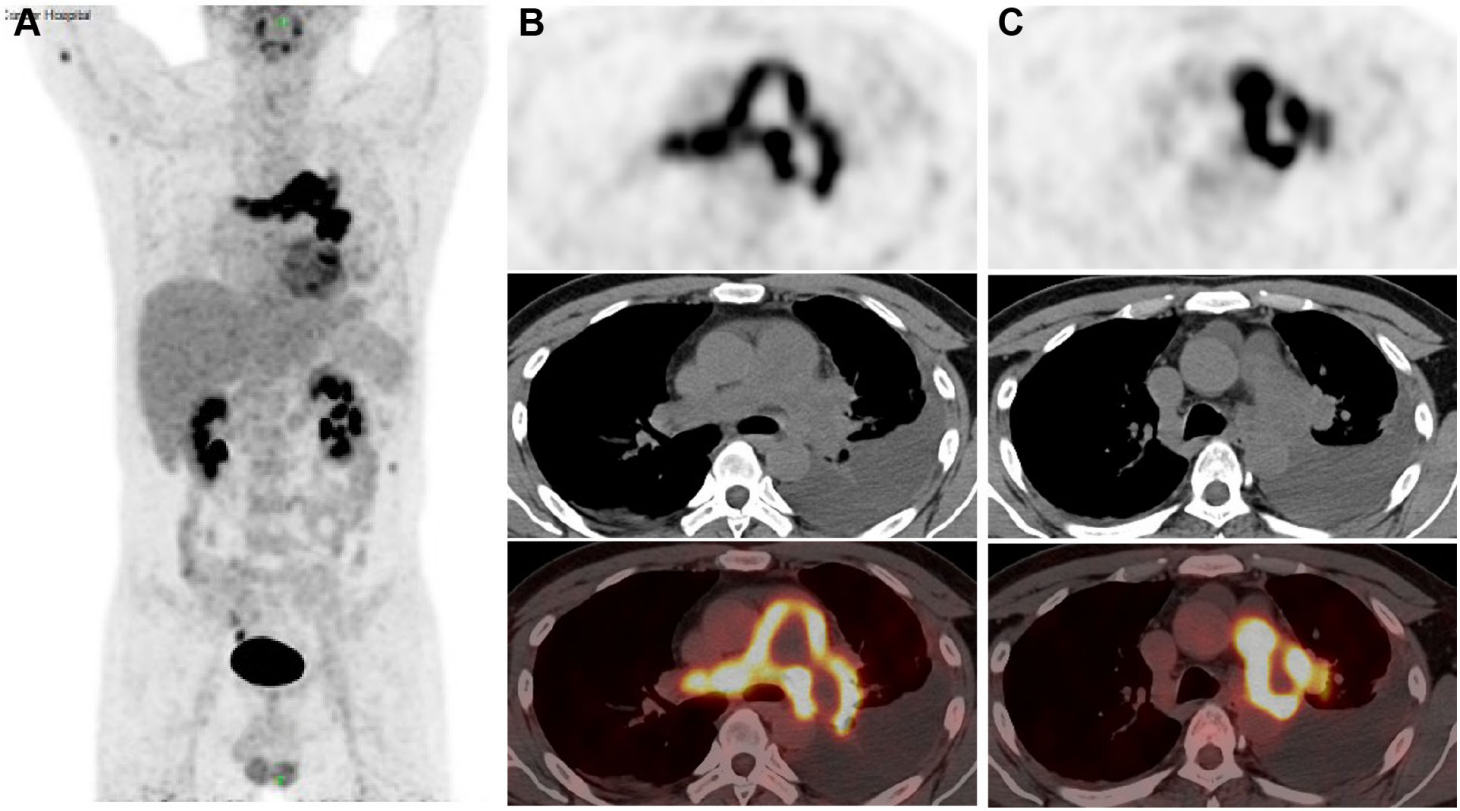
18F-FDG Positron Emission Tomography-Computed Tomography (PET-CT) images (A) The anteroposterior 3-dimensional maximum intensity projection image (MIP) demonstrated a hypermetabolic lesion in the left and right artery trunks, while abnormal FDG metabolic nodules were found in the right deltoid muscle and left transversus abdominis muscle; (B, C) irregular PAS lesion in the pulmonary artery wall, with increased FDG uptake (SUV max=12.7).
